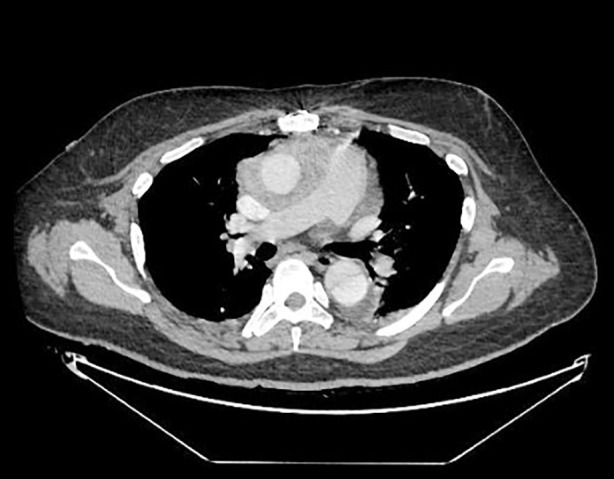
Computed tomography scan showing inflammation and thickening surrounding the ascending aortic graft.
